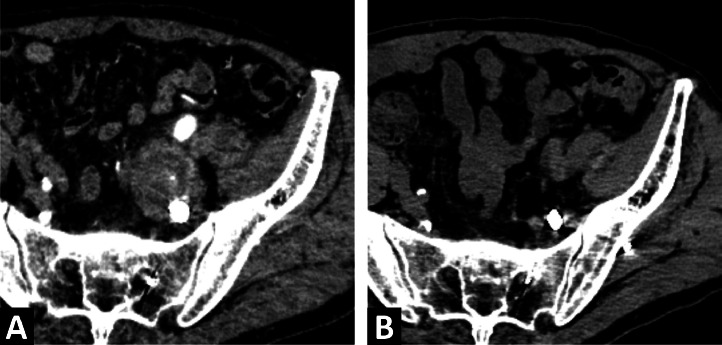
(A) Computed tomography angiography on day 6 after the procedure showing no enhancement of the aneurysm. (B) Three-year follow-up computed tomography angiography showing shrinkage of the aneurysm.
